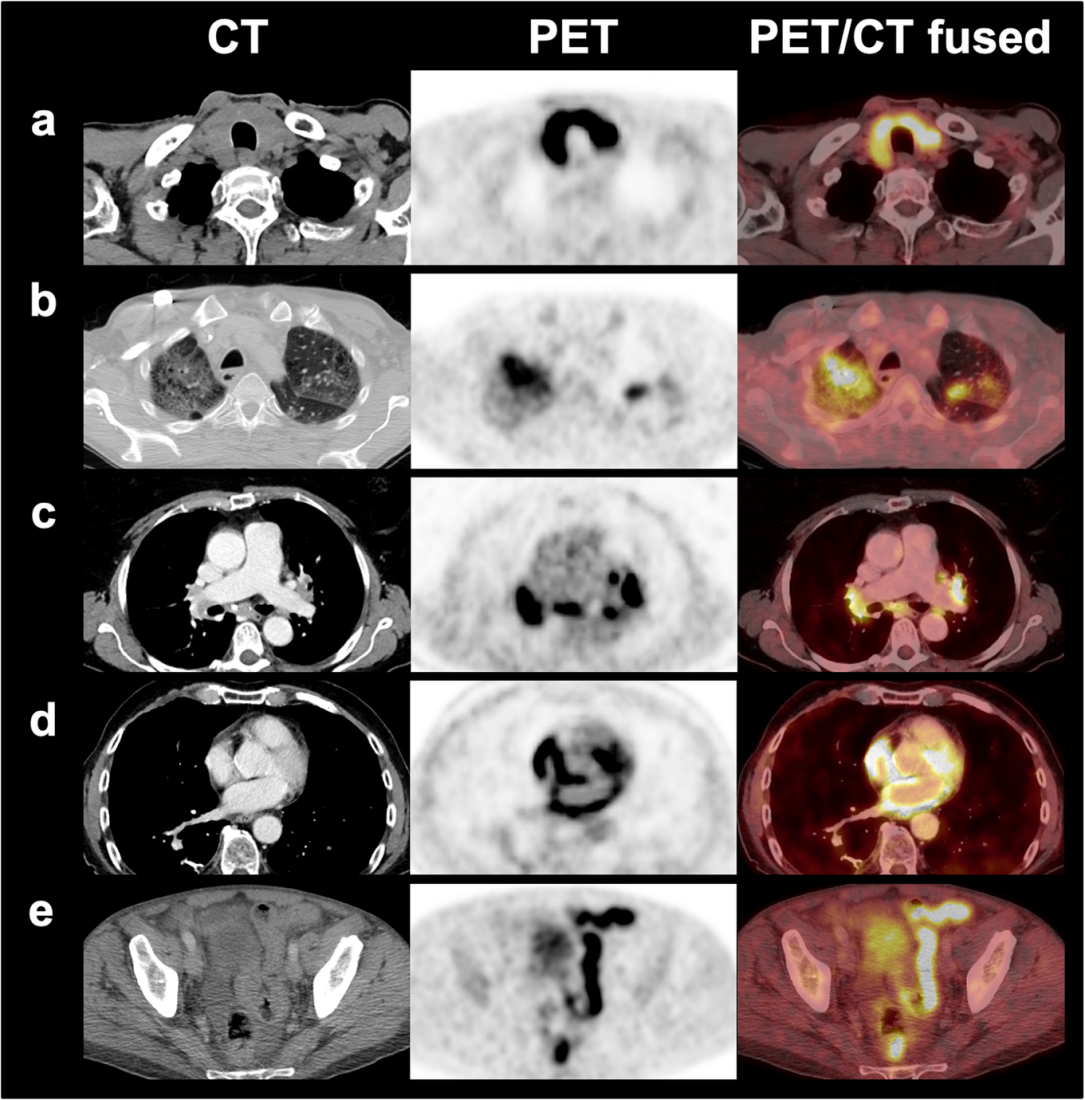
Examples of immune-related adverse events on positron emission tomography/computed tomography with intensely increased ^18^F-FDG uptake. a Thyroiditis. b Pneumonitis. c Sarcoid-like reaction. d Pericarditis. e Colitis
